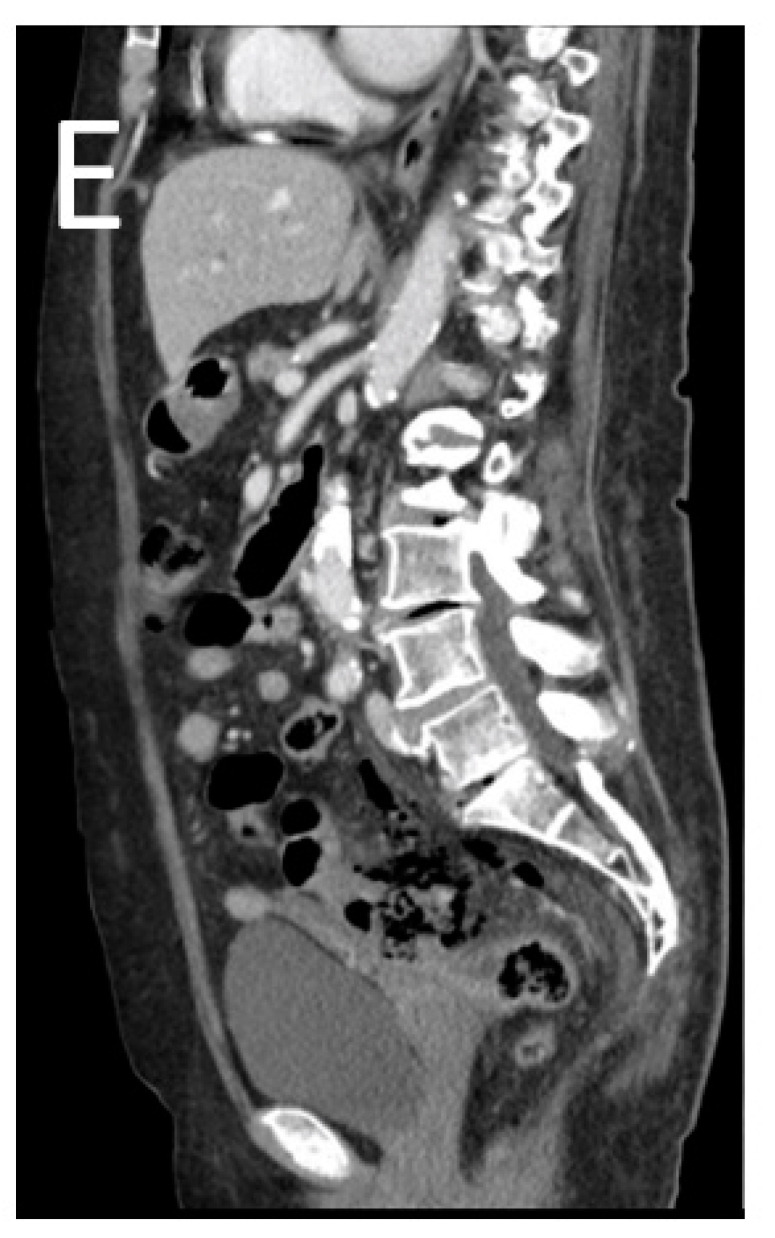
A 69-year-old female with a history of santol seed ingestion. Surgical diagnosis was full thickness of necrosis of the upper rectum with retained santol seed in the submucosal layer. CT shows retroperitoneal free air in the inframesocolic compartment (arrow in (A)), round hyperdense opacification in the middle of the fecal-like content adjacent to the involved upper rectum (arrow in (B)) and free air close to the involved upper rectum (C). Note the focal bowel wall disruption with abnormal thickness and enhancement of the involved upper rectum (D,E).
